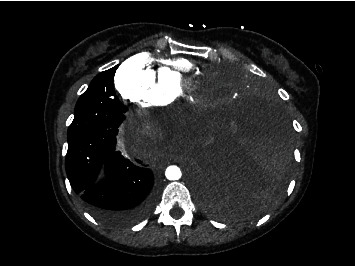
CT axial view arterial phase postcontrast large 17.9 × 17.6 × 18.3 cm heterogeneous mass centered within the left hemithorax, resulting in rightward mediastinal shift. An incidental right pleural effusion is present.
